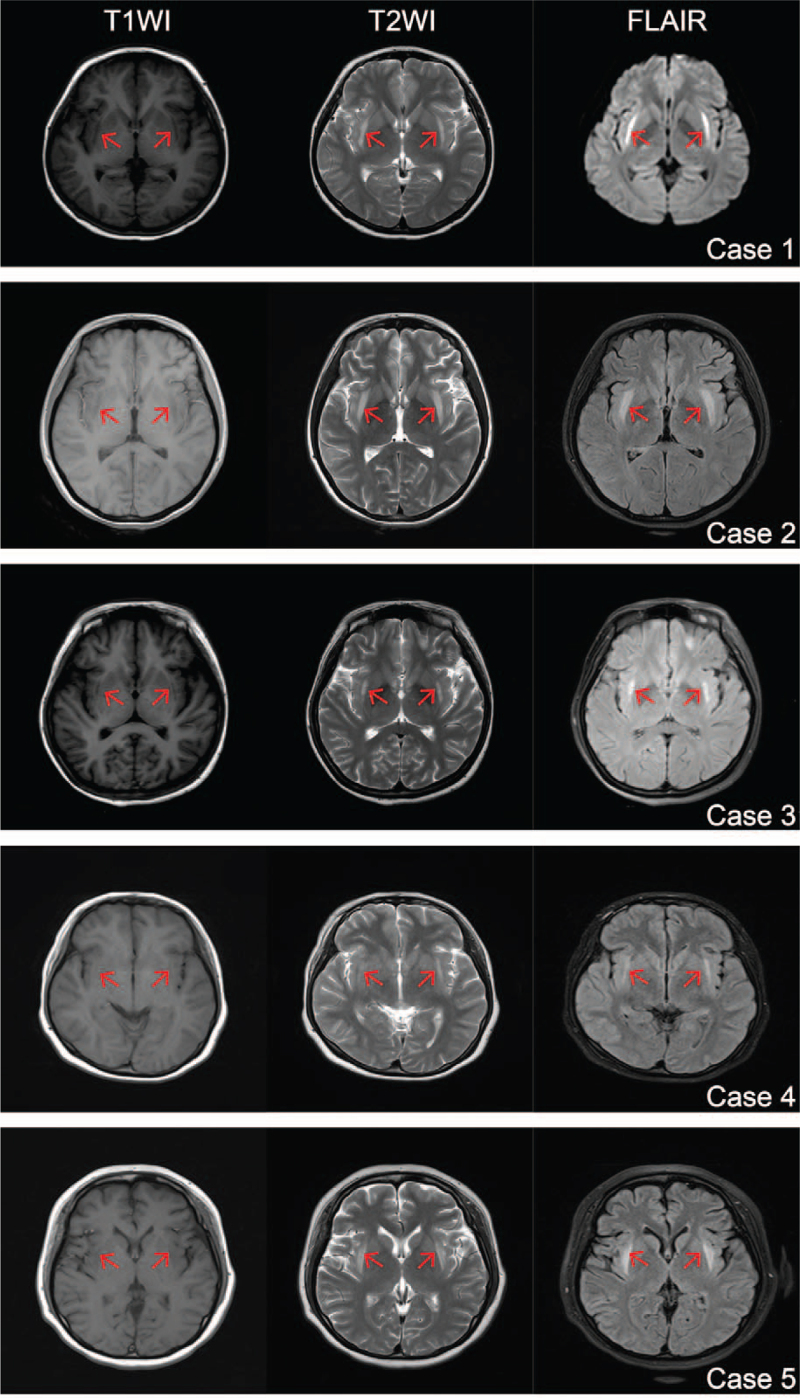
Magnetic resonance images of 5 cases. The claustrum of all patients showed symmetrical low signal lesions on T1WI, high signal lesions on T2WI, and FLAIR (red arrows). FLAIR = fluid-attenuated inversion recovery, T1WI = T1-weighted images, T2WI = T2-weighted images.
